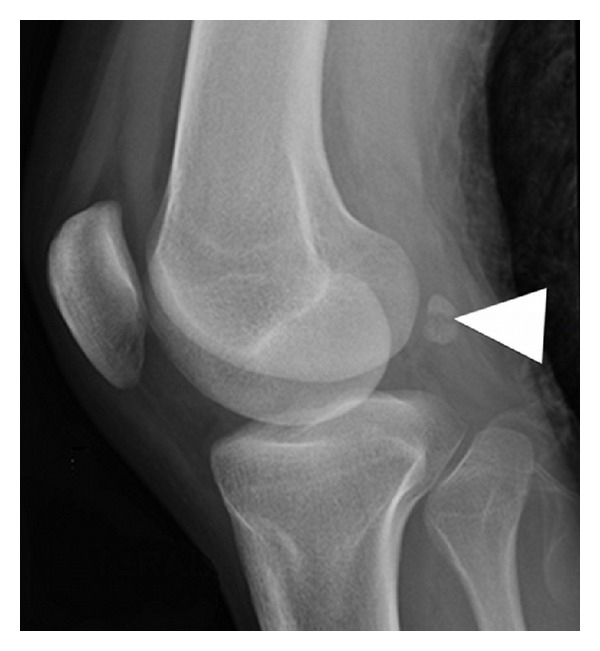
Lateral plain film of the left knee revealed a transverse radiolucent line with irregular borders across an ossified fabella (arrowheads) consistent with a complete fracture of the fabella.
